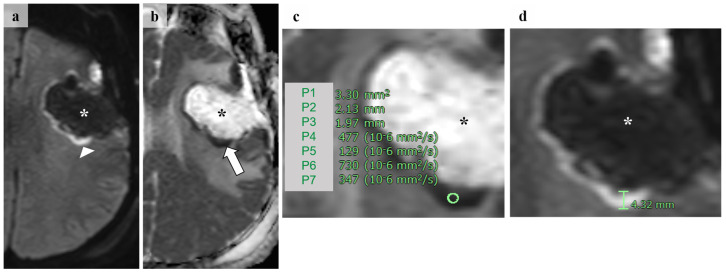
DWI hyperintensity thickness and ADC value measurement. A representative postoperative MRI demonstrates an area of restricted diffusion at the intraoperative PD laser irradiation site (indicated by the arrowhead and arrow) around the resection cavity (asterisk). (a) Diffusion-weighted image (DWI). (b) Apparent diffusion coefficient (ADC) map. (c) Enlarged view of the ADC map, with the circle indicating the region of interest (ROI). The measured parameters (P1–7) for the ROI are listed from top to bottom: area, major axis, minor axis, mean, standard deviation, maximum, and minimum. (d) Enlarged view of the DWI, with the line indicating the thickness of DWI hyperintensity.
